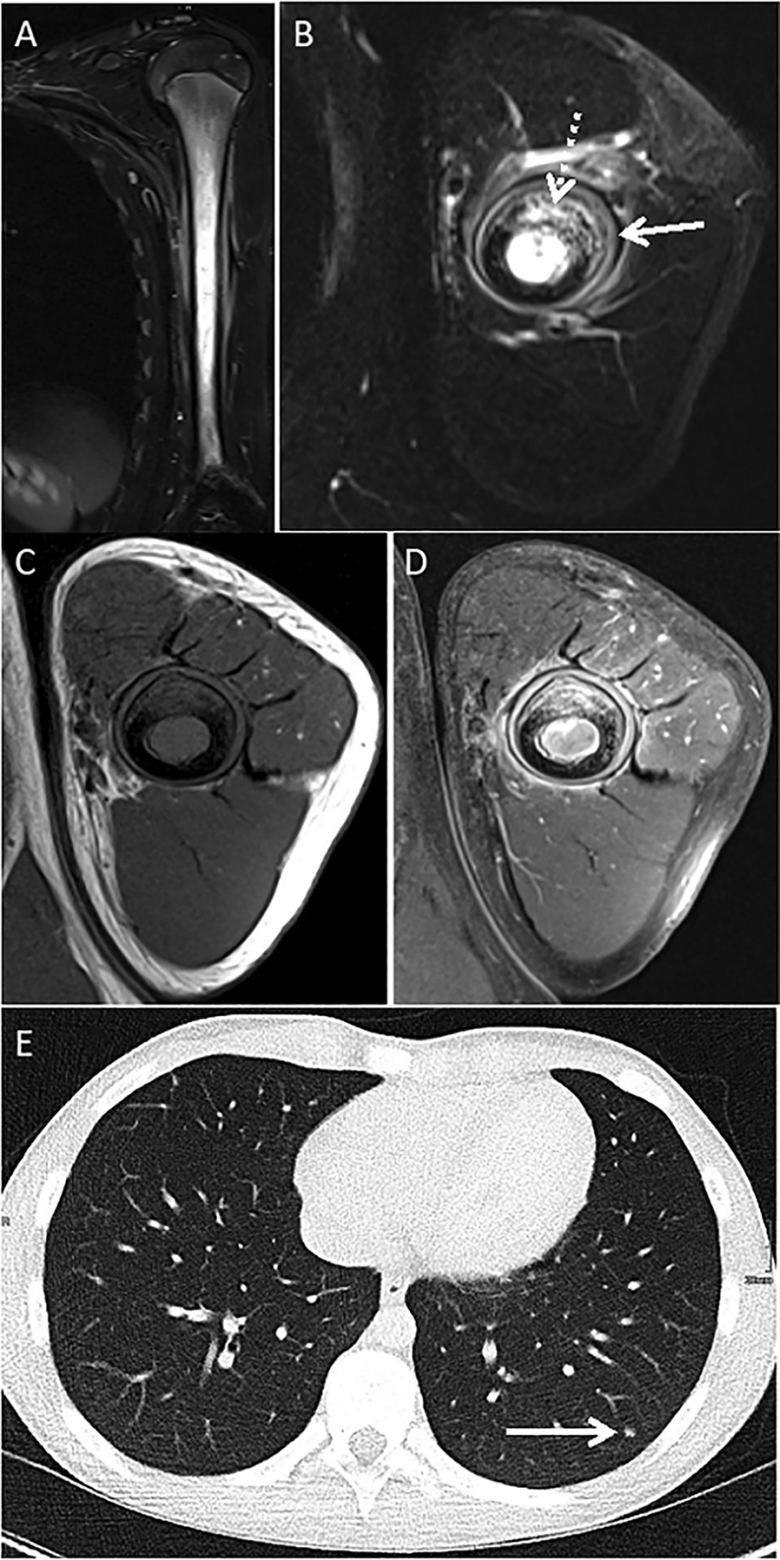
A 14‐year‐old, previously healthy boy developed intermittent pain of his left humerus. An x‐ray was taken six months after the onset of symptoms and revealed an osteolytic lesion. A subsequent MRI showed signs of bone inflammation, osteolysis, and circular bone formation (A–D). By the local pathology service, bone biopsies were interpreted as suggestive of high‐grade osteoblastic osteosarcoma and chest CT revealed the presence of pulmonary infiltrates. Six weeks after starting high‐dose polychemotherapy with methotrexate, doxorubicin, and cisplatin, following EURAMOS/COSS protocols (https://www.sciencedirect.com/science/article/pii/S095980491831534X), national reference pathology results raised doubts in relation to suspected malignancy. A third opinion from an international bone tumor reference center suggested repeating open biopsy, which was suggestive of CNO (chronic inflammatory infiltrates and bone sclerosis) in the absence of malignancy. As a result, the patient was referred to the local pediatric rheumatology service and successfully treated for CNO. (A–D) MRI of the left humerus is shown. (A) Coronal and (B) axial TIRM sequences show hyperintense lesions with cortical destruction (ventral, dotted arrow) with diffuse signal alterations affecting the bone marrow cavity. Circular periosteal bone formation (solid arrow) and limited perifocal soft tissue edema are shown. (C and D) T1 weighted sequences (axial) with (D) contrast enhancement in the bone marrow cavity, the destructed cortical bone, the periosteal new bone, and the perifocal soft tissue are shown. (E) Chest CT with three nodular (only one shown, arrow) opacities in the left lower pulmonary lobe (~5 mm in diameter) is shown. CNO, chronic nonbacterial osteomyelitis; CT, computed tomography; MRI, magnetic resonance imaging; TIRM, turbo inversion recovery magnitude.
